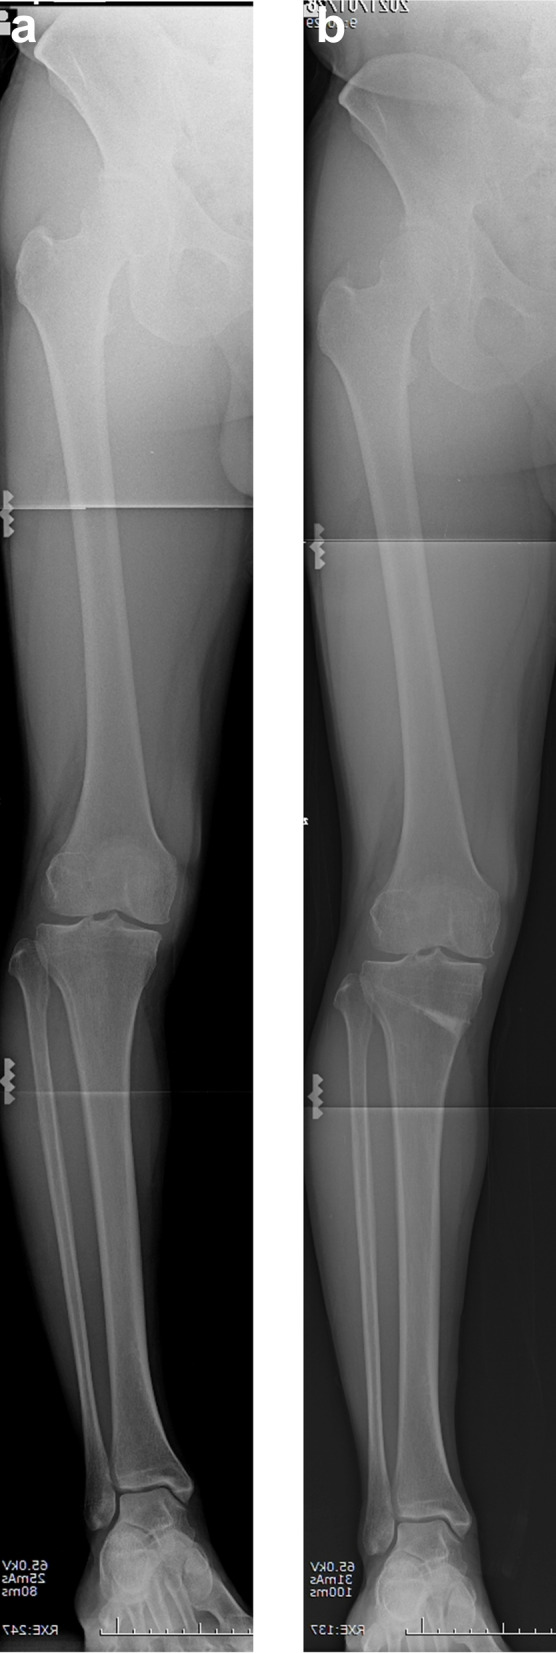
Pre- and post-operative hole bilateral standing whole-leg radiographs. a Preoperative radiograph, b Post-operative (final follow-up) radiograph
